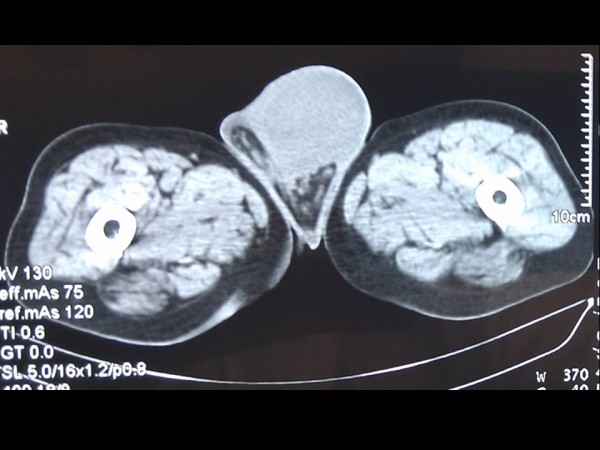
Scrotal computed tomography demonstrating a mass in the left hemiscrotum.
